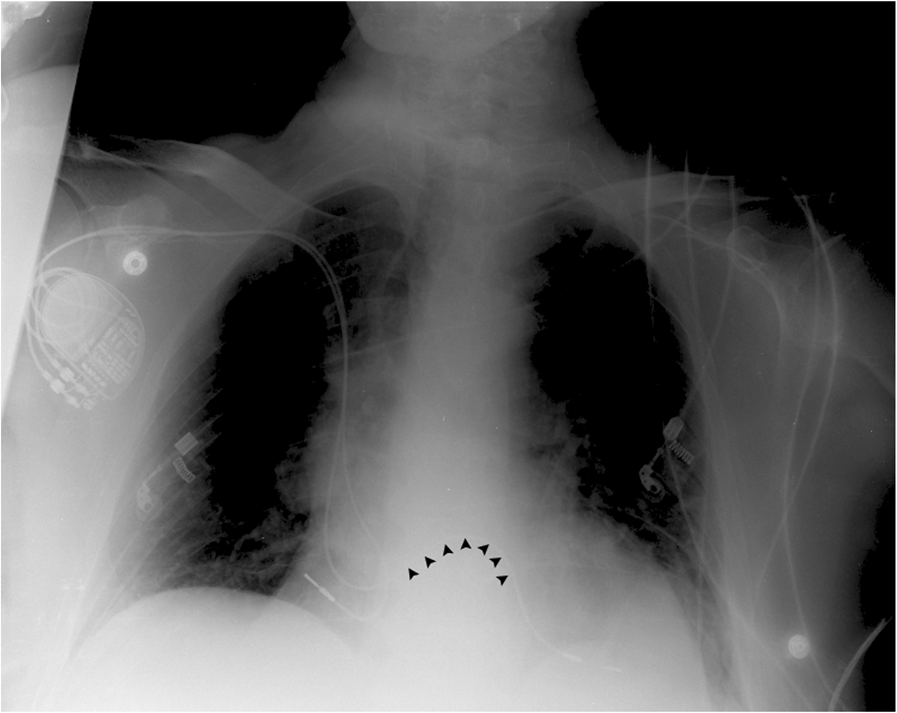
Chest X-ray. The ventricular pacemaker lead follows an unusual course (black arrows) due to its placement via a PFO into the left ventricle.
